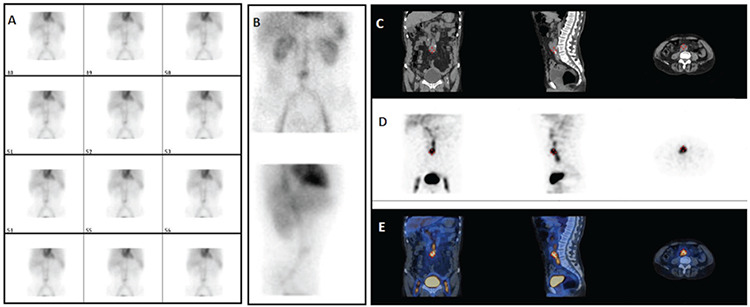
Increased uptake in the midline of the abdomen adjacent to the aorta was noted since the beginning of dynamic images of a 63-year-old male (A). In the late planar images, increased uptake in this area continued, whereas no other area suggested active bleeding in another region [(B) anterior and lateral planar images]. SPECT/CT imaging was performed to interpret this area more accurately with increased uptake. The CT component of SPECT/CT was consistent with the previously unknown aortic aneurysm [(C) CT, (D) SPECT, (E) fused SPECT/CT images in coronal, sagittal, and axial planes] SPECT/CT: Single photon emission computed tomography/computed tomography
